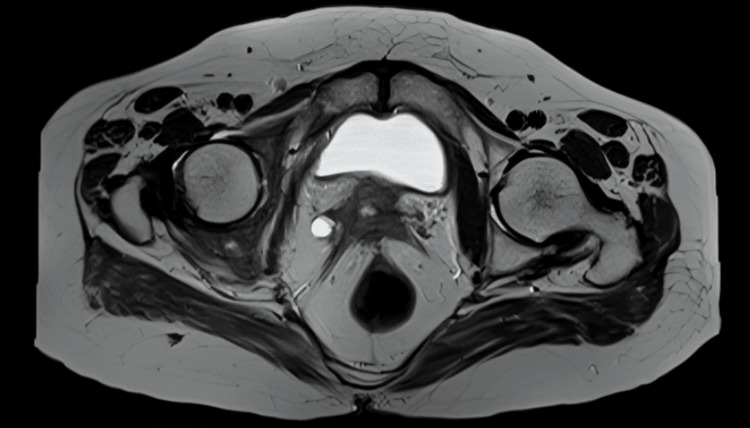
T2-weighted imaging MRI (axial view) demonstrating parametrial invasion and right ureteric invasion with consequent right hydroureteronephrosis
